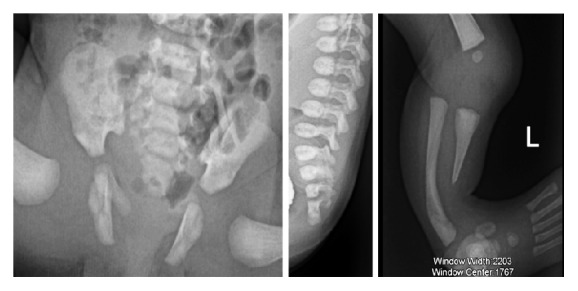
Radiographs centered on the anomalies of the bony structures (frontal view of the pelvis, lateral views of the lumbosacral spine and the left leg): VACTERL. Pubic diastasis, fusion of the last sacral vertebrae with agenesis of the coccyx, agenesis of the distal half of the tibia with fibular curvature, and an equinovarus deformation of the left foot.
